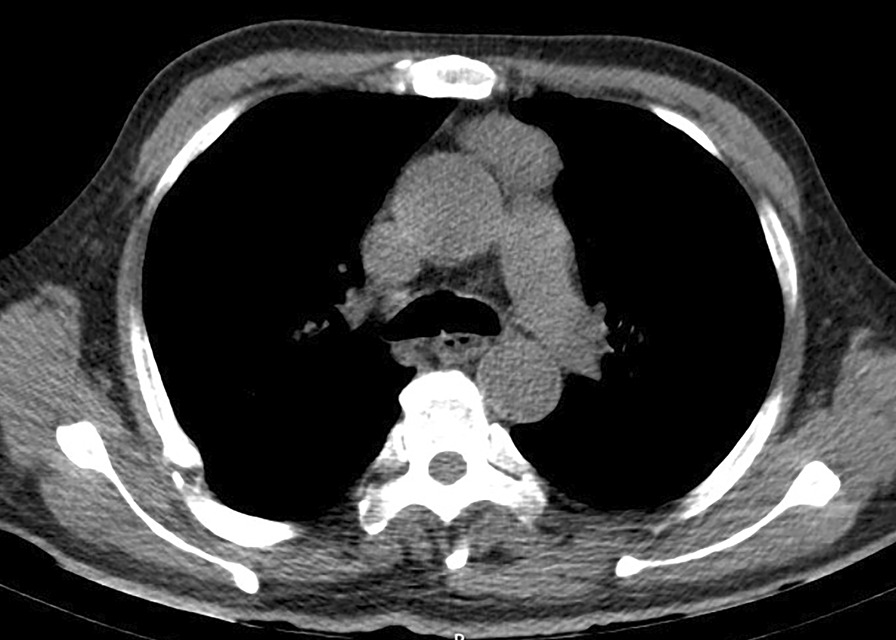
CT scan showing an anterior mediastinum soft tissue shadow
